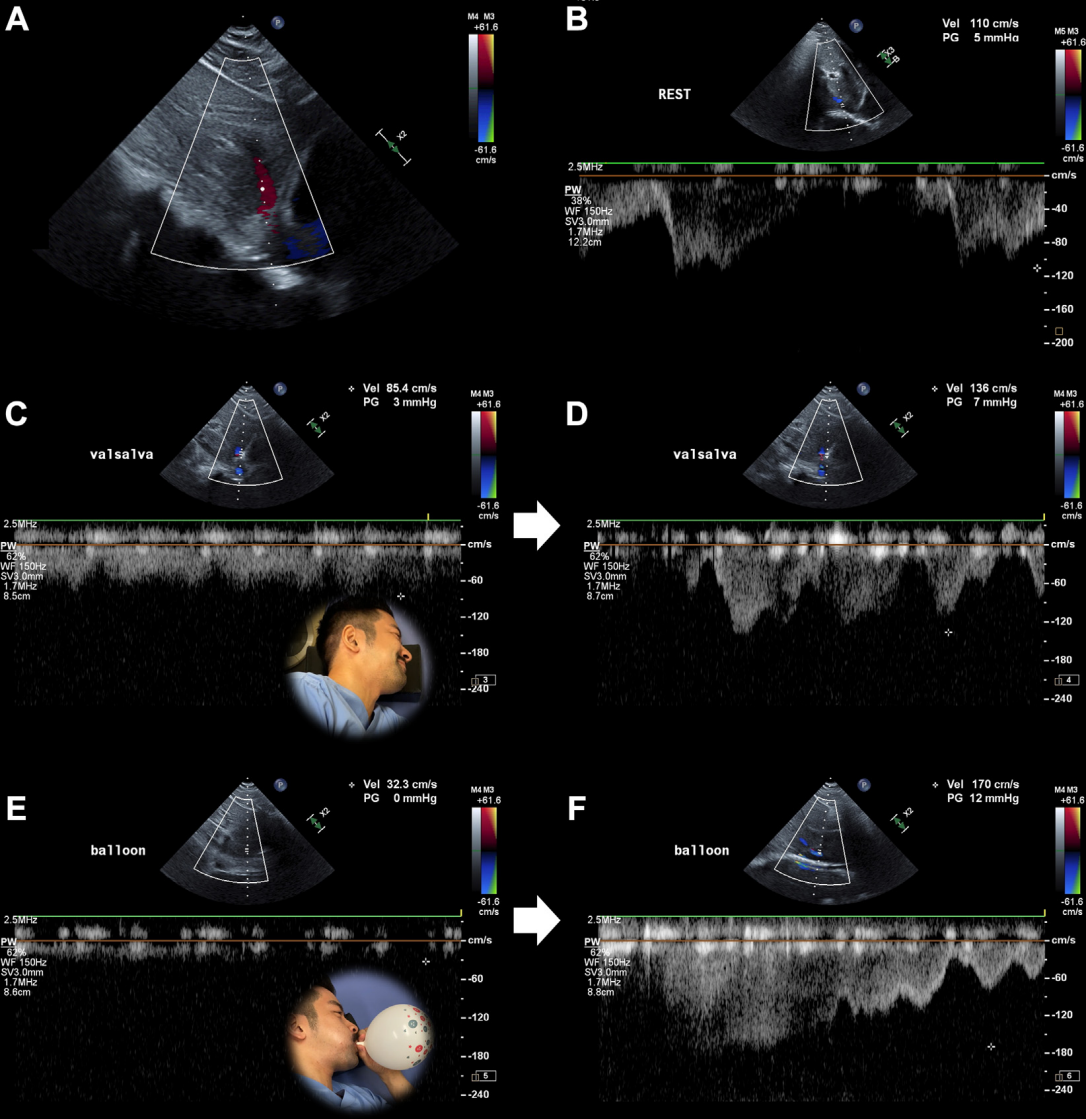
Color Doppler and Pulse-Wave Doppler of Hepatic Vein Flow (A) Color Doppler image of the hepatic vein. (B) Pulse-wave Doppler measurement of hepatic vein flow at rest shows a maximum flow velocity (MFV) of 110 cm/s toward the inferior vena cava. During the conventional Valsalva maneuver, (C) the MFV was reduced to 85.4 cm/s and (D) increased to 136 cm/s after release. With balloon inflation, (E) the MFV of the hepatic vein was further reduced to 32.3 cm/s and (F) increased to 170 cm/s after release (inspiration phase), indicating a substantial increase in hepatic vein flow compared with that of the conventional Valsalva maneuver.
